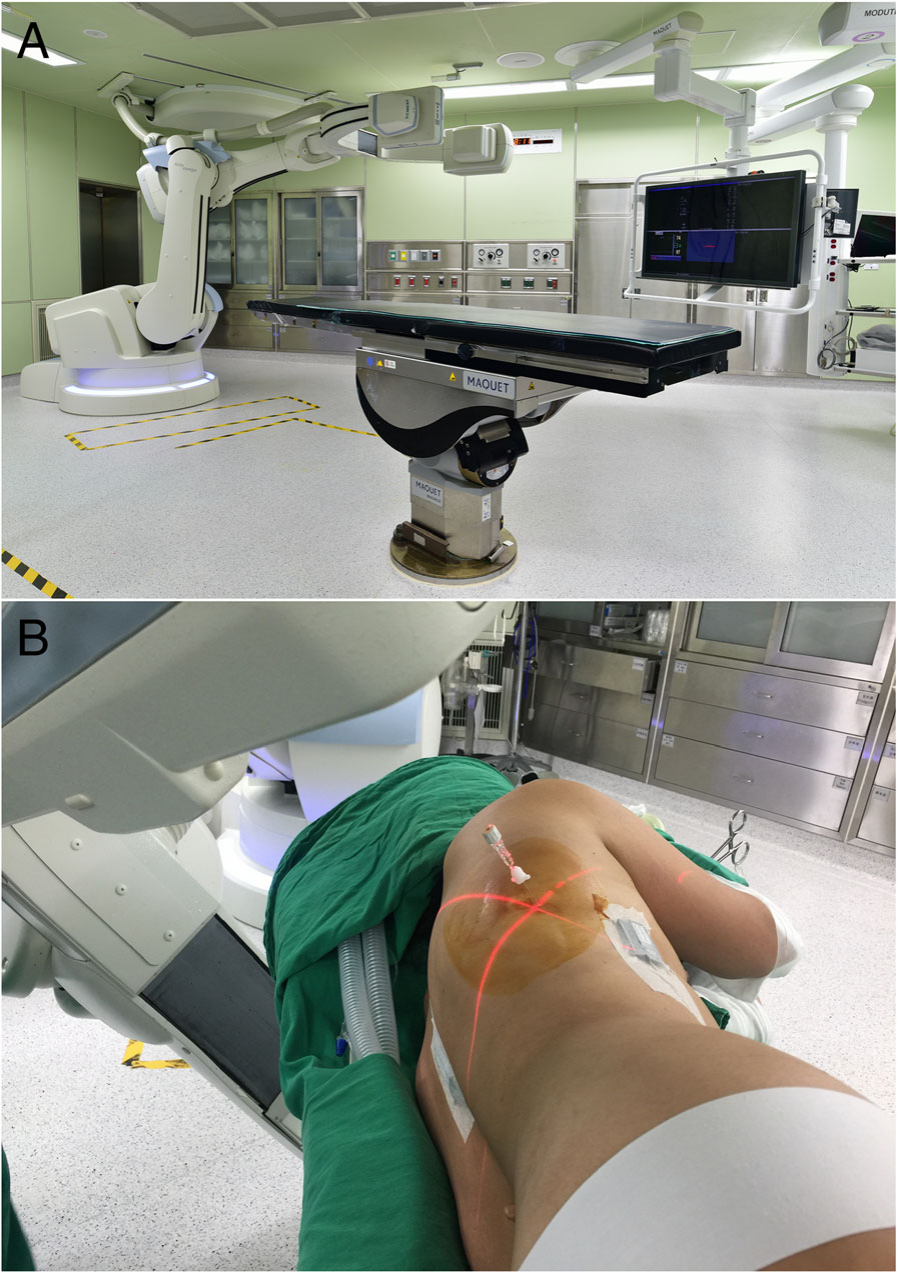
a Hybrid operating room equipped with a cone-beam CT apparatus (ARTIS zeego; Siemens Healthcare GmbH, Erlangen, Germany) and a Magnus surgical table (Maquet Medical Systems, Wayne, NJ, USA). b The needle entry point and angulation were visualized by projecting a laser-targeting cross onto the patient’s surface
